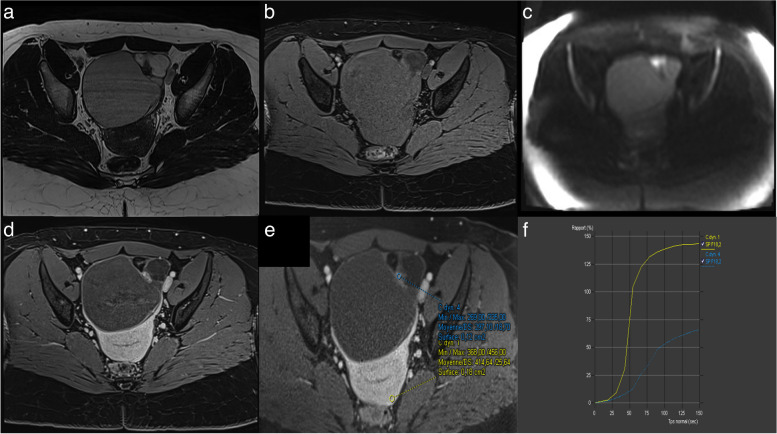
Example of mature cystic teratoma. a Axial T2-weighted sequence without fat saturation. b Axial unenhanced T1-weighted Water sequence. c Axial DWI scan (*b*: 1200 s/mm^2^). d Axial late-gadolinium T1-weighted sequence. e, f DCE T1-weighted sequence with corresponding curve of the external myometrium: yellow curve and Rokitansky protuberance: purple curve corresponding to a type I curveEndosalpingeal folds (Fig. 11). Another common cause of false positives is the misinterpretation of endosalpingeal folds as papillary projections, especially in the context of pelvic inflammatory disease (PID). PID is typically associated with inflammatory processes, leading to thickening, and marked enhancement of the fallopian tube wall and endosalpingeal folds. A study by Thomassin-Naggara et al. reported that 11 out of 12 PID cases were incorrectly scored as O-RADS MRI category 4 or 5 because the TIC analysis was performed by placing a region of interest (ROI) on endosalpingeal folds or thickened wall [7]. Radiologists should exercise caution and consider all imaging findings, such as premenopausal status, tubular shape, inflammatory changes in adjacent structures, and the presence of pus within the lesion. Pus can be recognized as high signal intensity on high-b-value DWI with a corresponding low signal intensity on the ADC map.
